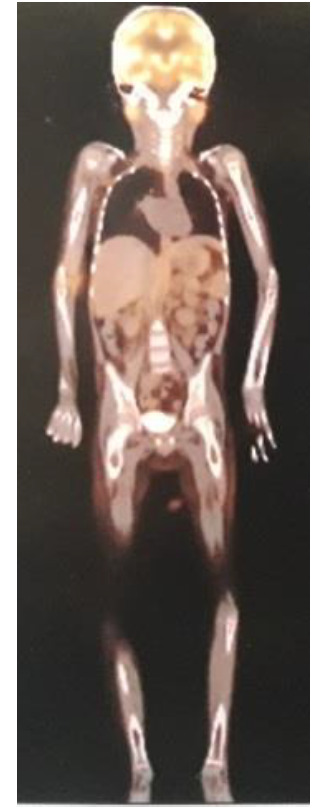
Result of fluorodeoxyglucose positron-emission tomography (FDG/PET) scan after four months of antifungal treatment (Despite primary multiorgan involvement in the lung, liver, and spleen, no evidence of metabolically active lesion was noted throughout the body in the follow-up FDG/PET scan.)
